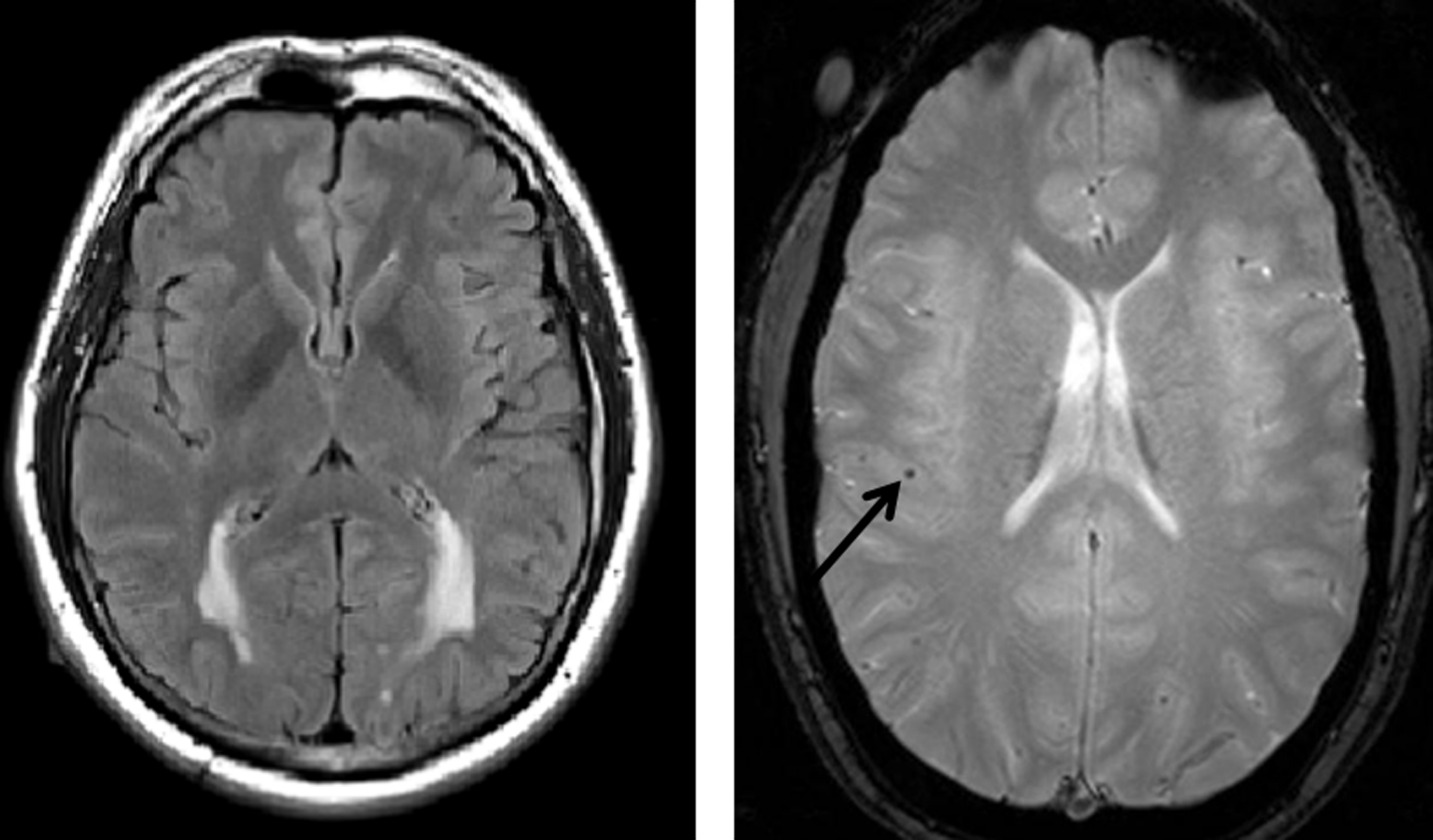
Examples of WMH and microbleeds. Left: T2-weighted FLAIR images from a mutation carrier with prominent posterior WMH, approximately 5 years prior to symptom onset. Right: example of microbleed (arrow) on a gradient echo MRI scan.
